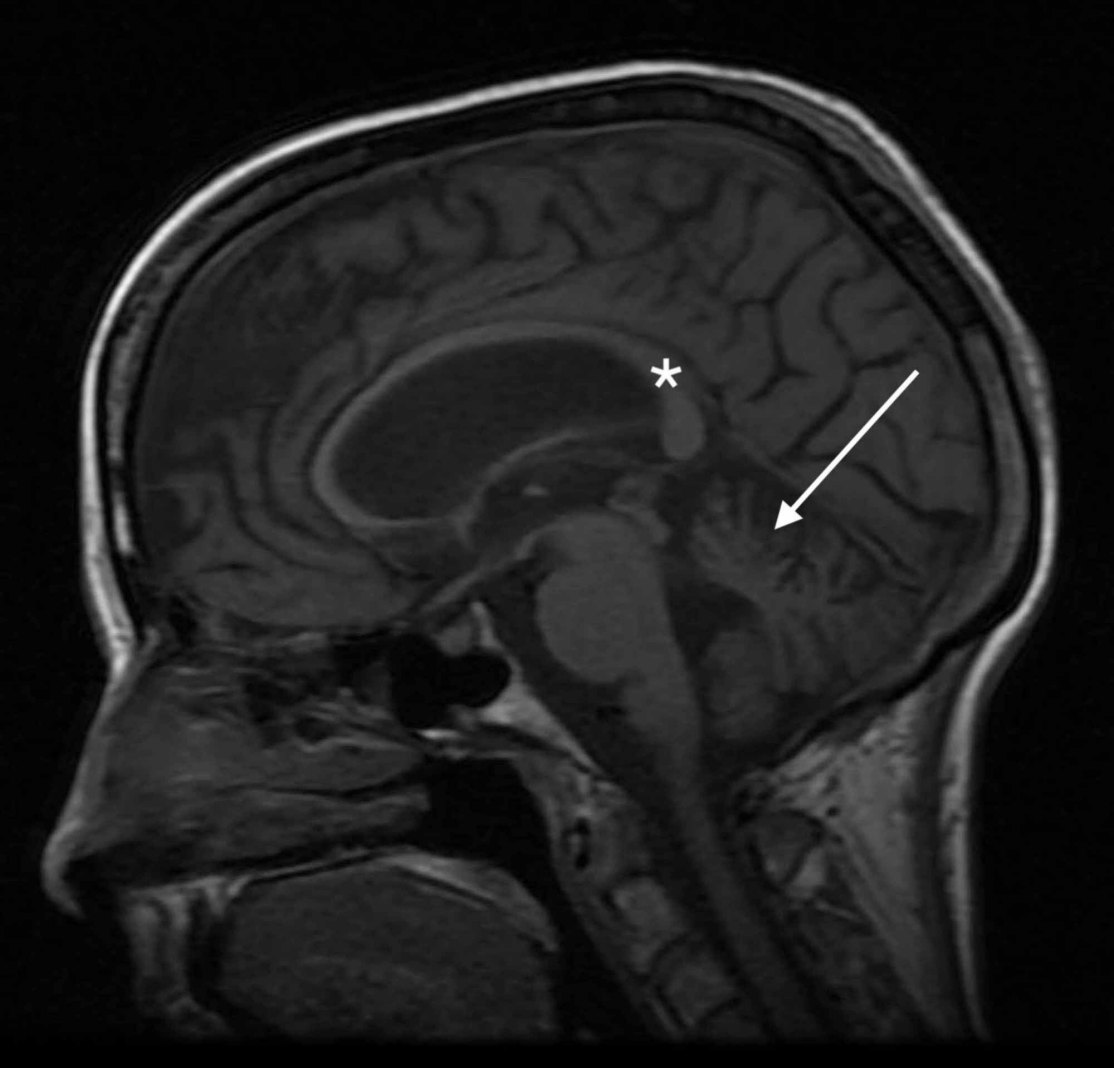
Mid-sagittal T1-weighted MRI of the head Mid-sagittal T1-weighted MRI of the head shows cerebellar atrophy (arrow) and a normal corpus callosum (star)
